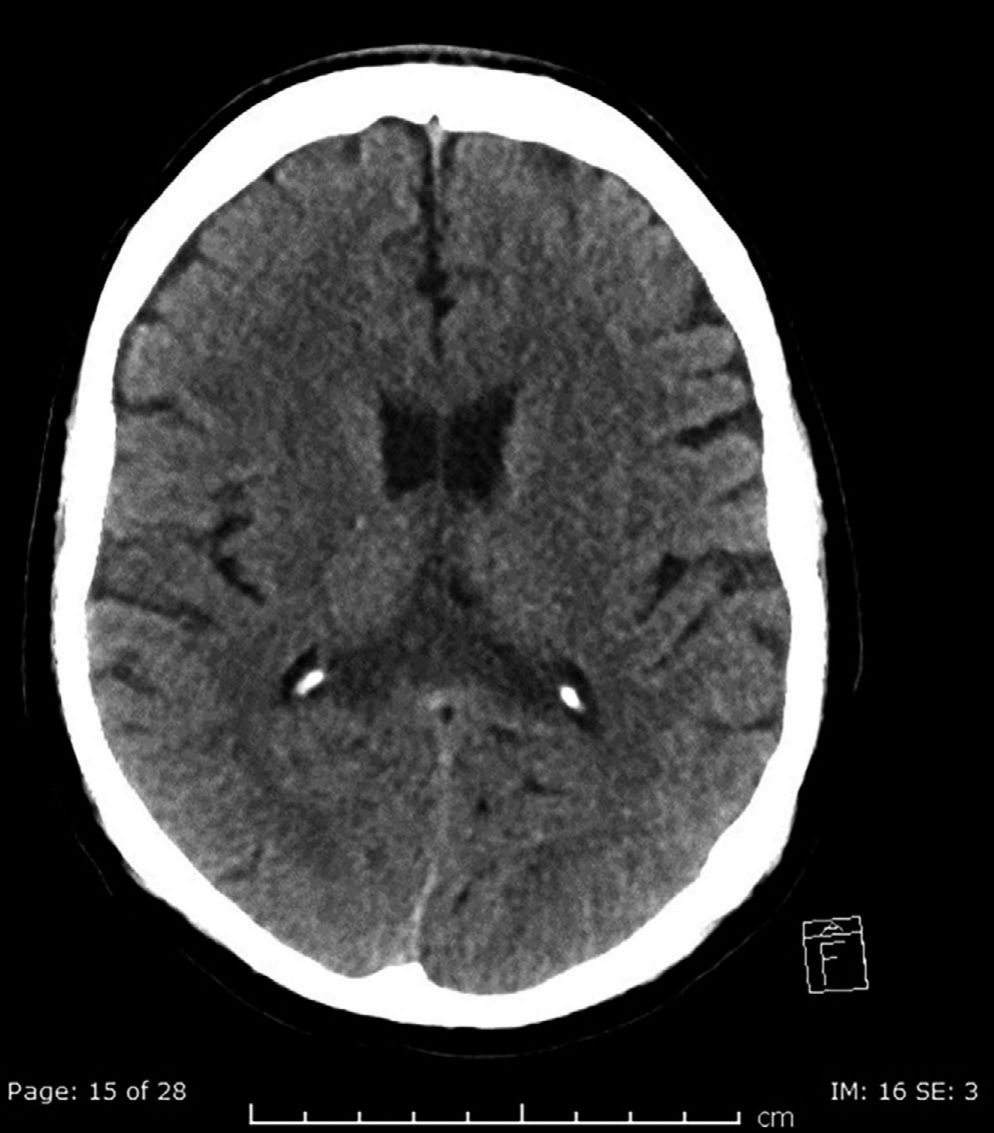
Follow‐up axial CT head image after 1 week shows resolution of the hematoma with residual hypodensity in the splenium
